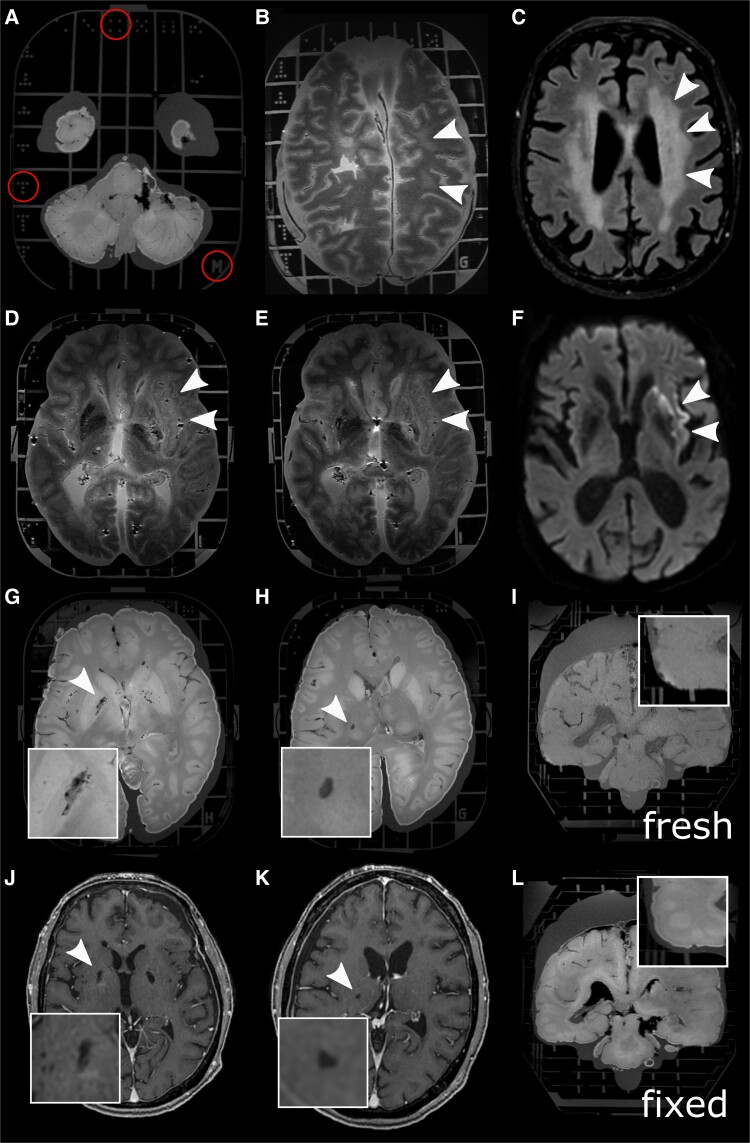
Whole-brain MRI using the Brainbox. the Brainbox has an imprinted 3D coordinate system with Latin letters in Z direction and unequivocal dots in X and Y direction (A). Whole-brain post-mortem MRI from a patient with X-linked adrenoleukodystrophy shows T2w hyperintensities in the deep white matter (B, white arrowheads), similar to the premortem T2w imaging (C). T2w hyperintense, i.e. demarcated stroke in the vascular territory of the middle cerebral artery affecting the basal ganglia, the internal capsule and the insula, in fresh (D, white arrowheads) and after 3 weeks of formaldehyde fixation (E), similar to the diffusion restriction in premortem MRI (F). Also, smaller imaging features such as EPVS in the basal ganglia (G and J, premortem T1w image) or a lacunar stroke (H and K, premortem T1w scan) are conspicuous using our high-resolution MRI protocol of the whole human brain in the Brainbox (inlets with higher magnification). Brains which were fixed for 2–3 weeks show volume decrease (inlets with higher magnification of the temporal region) (I and L). Abbreviations: MRI, magnetic resonance imaging; T1w, T1-weighted; T2; T2-weighted.
